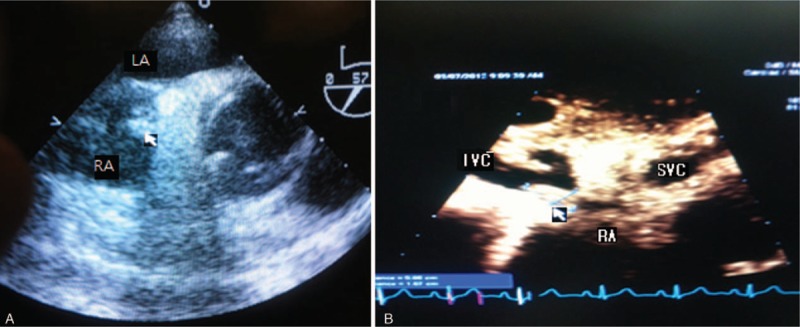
Transesophageal echocardiography finding. (A) In the midesophageal short axis view, the arrow indicates significant mass in RA. (B) In the bicaval view, the arrow indicates significant mass in IVC and RA. IVC = inferior vena cava, LA = left atrium, RA = right atrium, SVC = superior vena cava.
